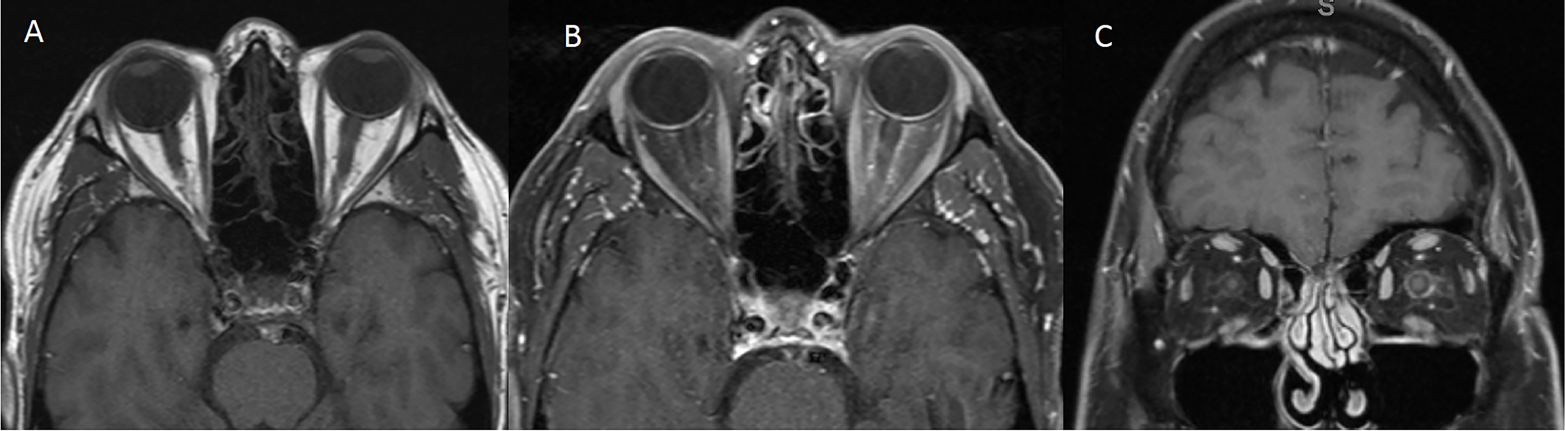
MRI of the orbits [(A) T1 Axial without contrast, (B) T1 Axial with contrast, (C) T1 Coronal with contrast] showing edema of the intraorbital and intracanalicular left optic nerve and associated enhancement of the intracanalicular segment with gadolinium.
